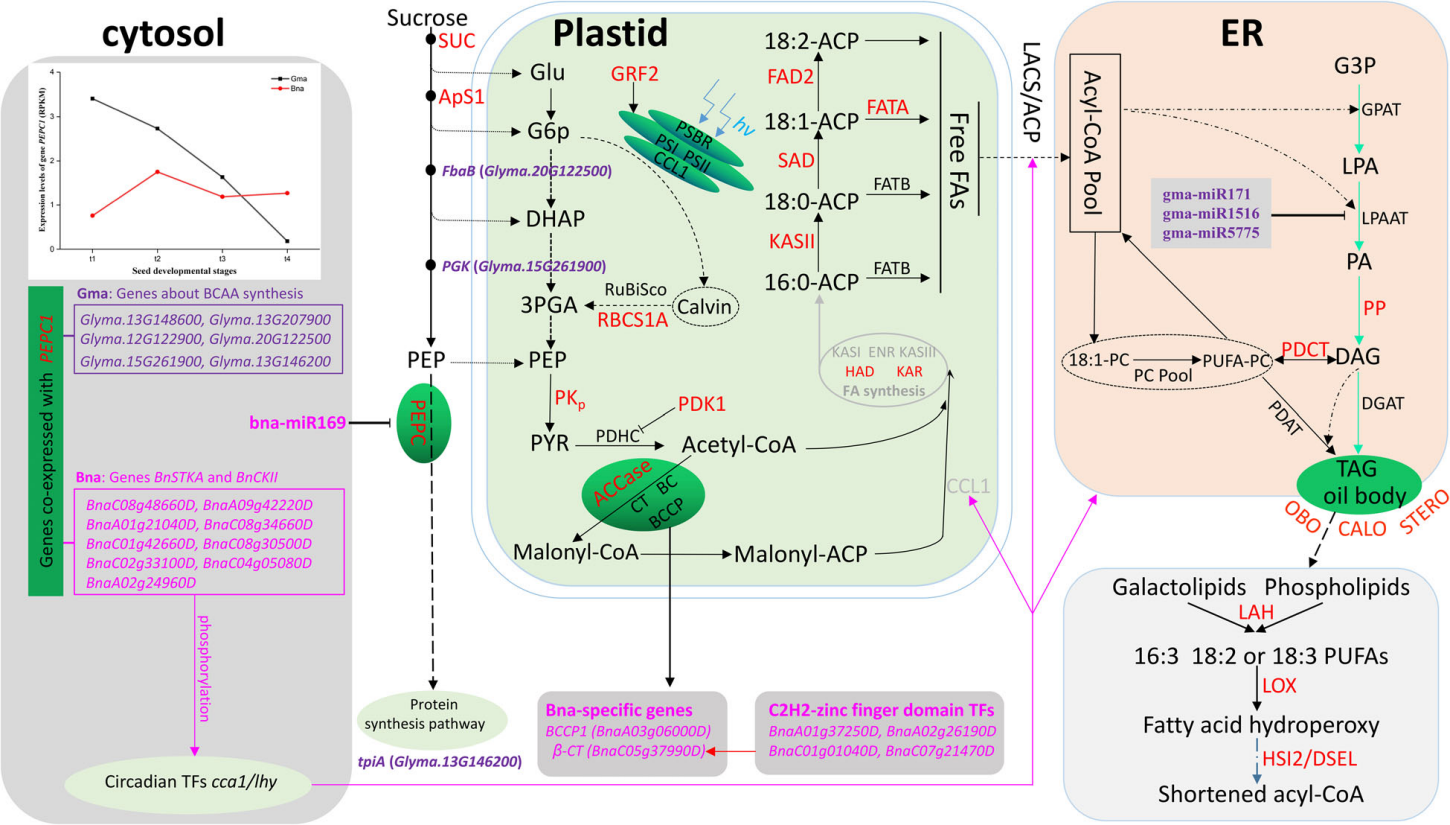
Molecular mechanisms for the difference of seed oil content between soybean and rapeseed. Candidate genes contributed to the differences of seed oil content between soybean and rapeseed obtained in the study were marked with red color. *GRF2* and *RBCS1A*: photosynthesis; *PGK*, *ApS1*, *SUC*, *PEPC* and *PKp*: carbon metabolism from sucrose to pyruvate; *PDK1*, *ACCase*, *KASII*, *HAD*, *KAR*, *FATA*, *SAD* and *FAD2*: in de novo fatty acid biosynthesis; *PAP* and *PDCT*: TAG synthesis; *OBO*, *CALO* and *STERO*: oil-body protein genes; *LOX*, *LAH*, *HSI2* and *DSEL*: oil degradation genes. Among these candidate genes, *BCCP1* (*BnaA03g06000D*) and *β-CT* (*BnaC05g37990D*) in heterogeneous acetyl-CoA carboxylase (ACCase) are rapeseed-specific genes, and *β-CT* is positively regulated by four transcription factors (*BnaA01g37250D, BnaA02g26190D, BnaC01g01040D* and *BnaC07g21470D*). The gene expression of *PEPC1* in rapeseed is putatively inhibited by bna-miR169, while *LPAAT* in soybean putatively inhibited by gma-miR171, gma-miR1516 and gma-miR5775 in triglyceride synthesis. The pink genes are speculated related specifically to high seed oil content of rapeseeds, and the purple speculated specifically related to high seed protein content in soybean, which were both identified by *PEPC* co-expression network analysis. Soybean genes participated in Branched-Chain Amino Acid (BCAA) synthesis may contribute to seed high protein content by adjusting the flow of PEP and downstream protein biosynthesis. Rapeseed genes *BnSTKA* and *BnCKII* are likely to promote the triglyceride synthesis by phosphorylating circadian TFs cca1/lhy and thus increase the seed oil content of rapeseed
